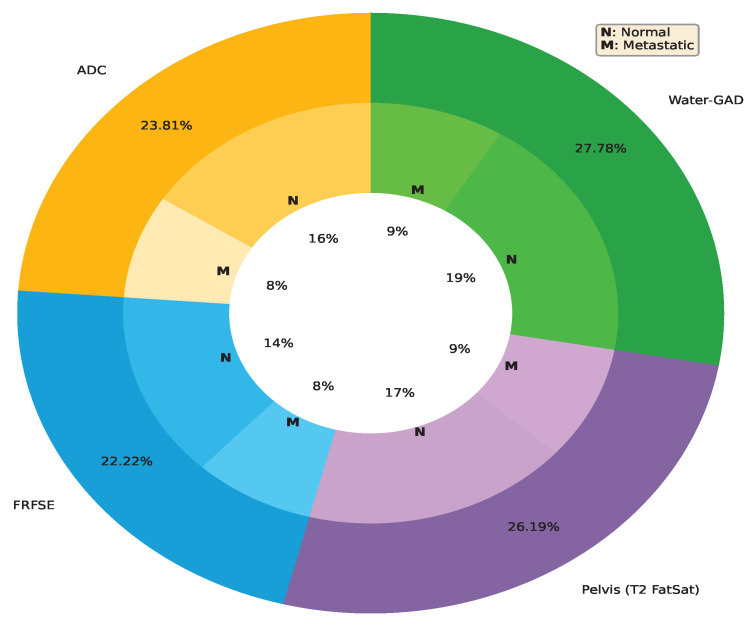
The distribution of normal and metastatic samples in each of the four MRI sequences, namely ADC, FRFSE, Water-GAD and Pelvis (T2 FatSat). The percentages in the inner circle represent the number of samples under each label–sequence combination as a percentage of the total number of samples present in the entire dataset, whereas the percentages in the outer circle represent the distribution of images across the four MRI sequences.
